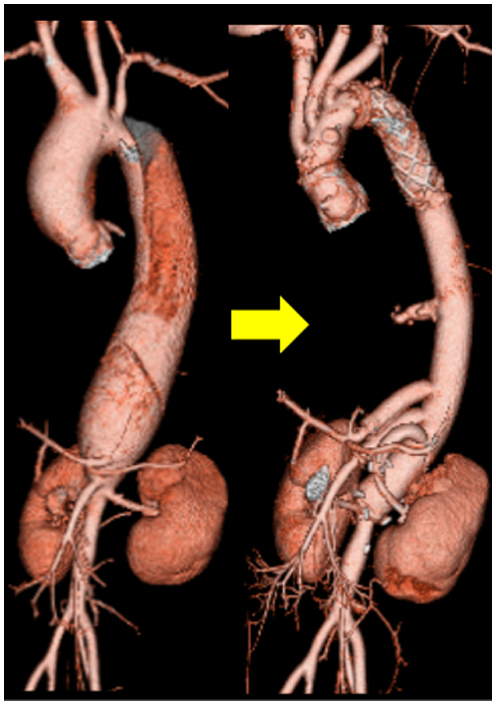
Preoperative and postoperative computed tomographic angiography.
